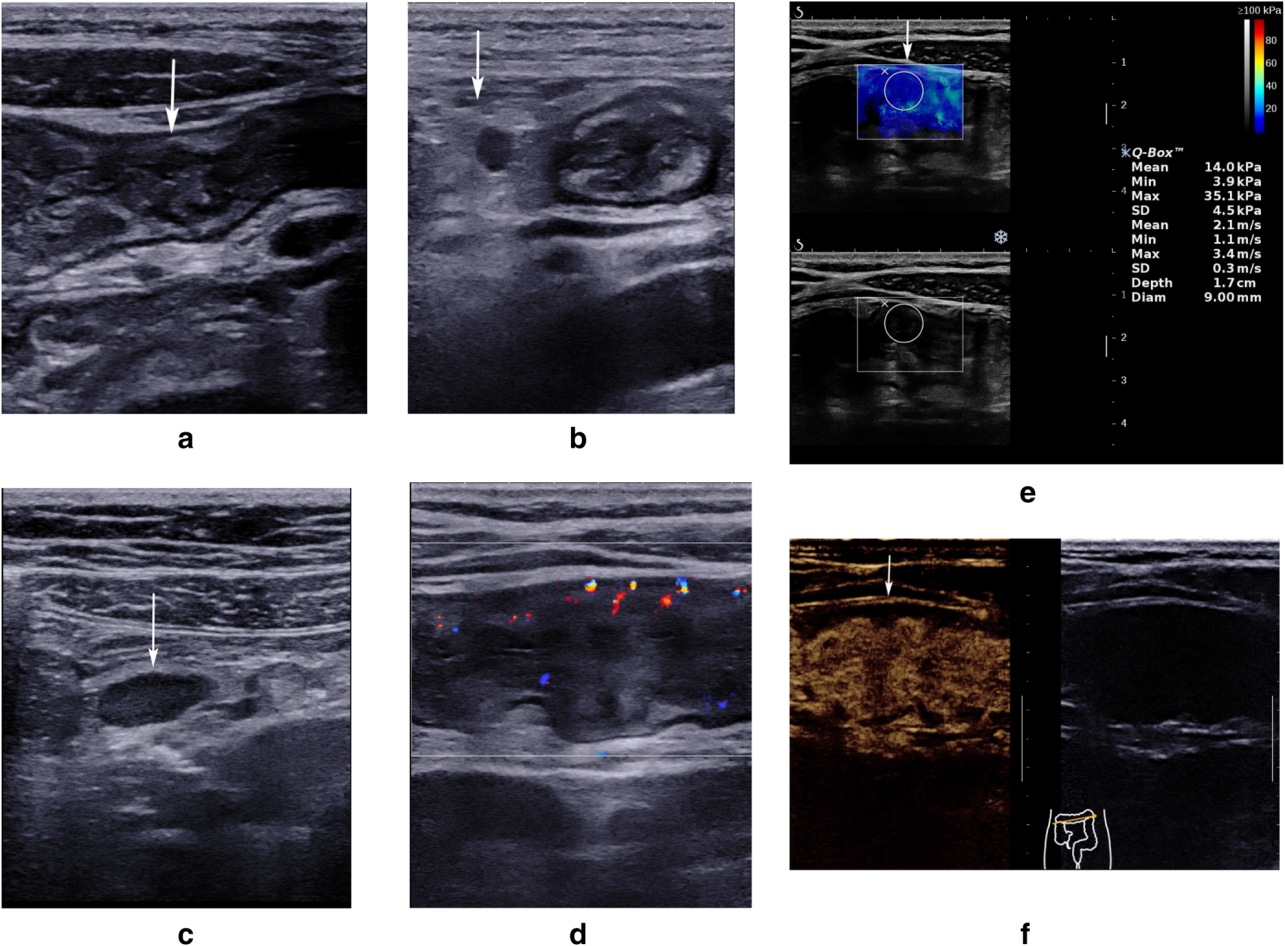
Examples of ultrasonic findings. Male, 18y, recurrent gastrointestinal bleeding for 1 year, CDAI: 336. (a) White arrow indicates thickening of the colon wall. (b) White arrows indicate mesenteric fat thickening. A lymph node can be detected in the thickened mesenteric fat. (c) White arrow indicates mesenteric lymphadenopathy. (d) The vascularization in the colon wall was scored to be Limberg II. (e) White arrow indicates that the anterior wall of the colon is soft, and the shear wave elastography of the anterior wall is homogeneous blue with a mean SWV of 2.1 m/s. Figure 1f. White arrow indicates a transmural homogeneous enhancement (TE homogeneous), and stratification of the intestinal wall still remains (SE presence).
